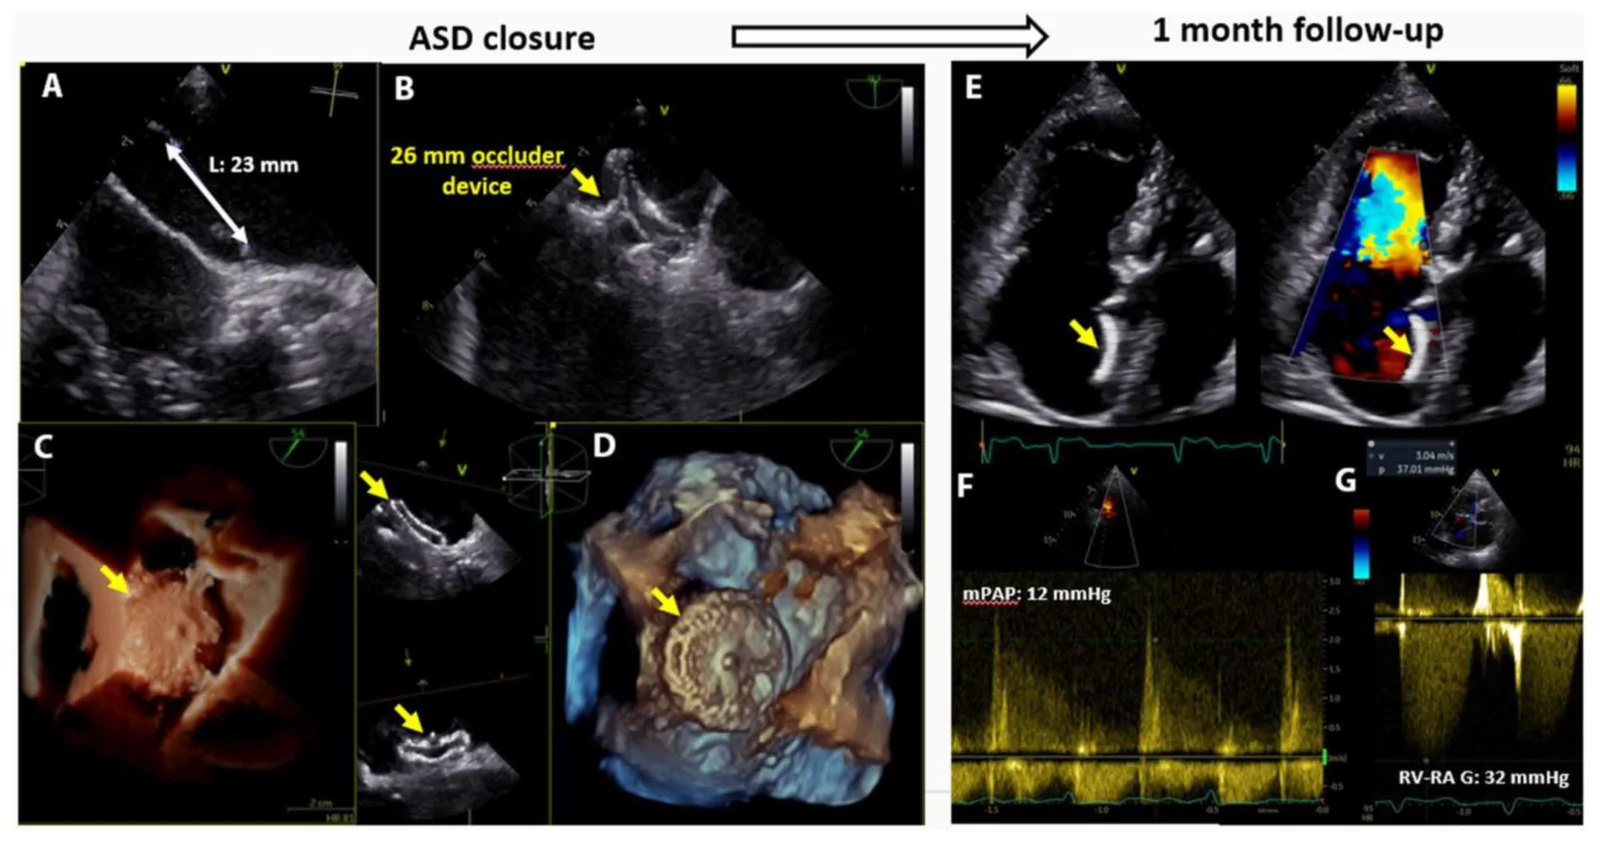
Percutaneous atrial septal defect (ASD) closure and follow-up evaluation. (A) Pre-procedural planning: modified bicaval TOE view showing a maximal ASD diameter of 23 mm; (B) Intraprocedural positioning of the occluder device; (C,D) Three-dimensional TOE echocardiographic reconstruction after successful deployment of a 26-mm occluder device; (E) Follow-up at 1 month demonstrating a well-seated device with a small residual shunt; (F,G) Significant improvement in PH (estimated mPAP: 12 mmHg).
